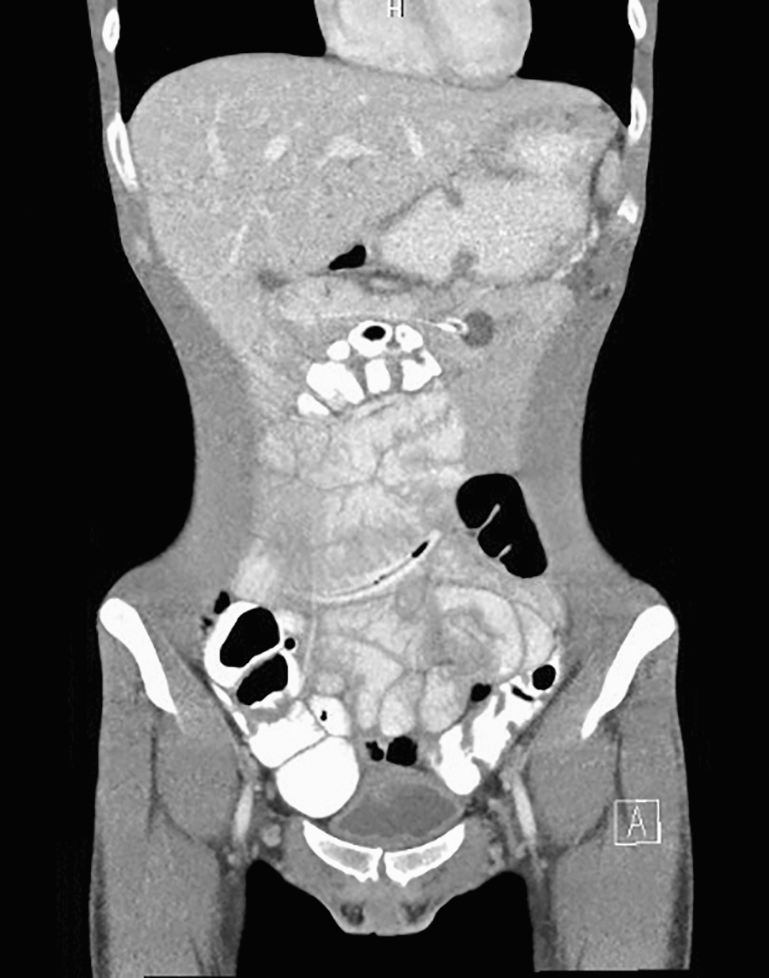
Coronal computed tomography demonstrating resolution of small bowel obstruction following balloon deflation and gastric decompression.
